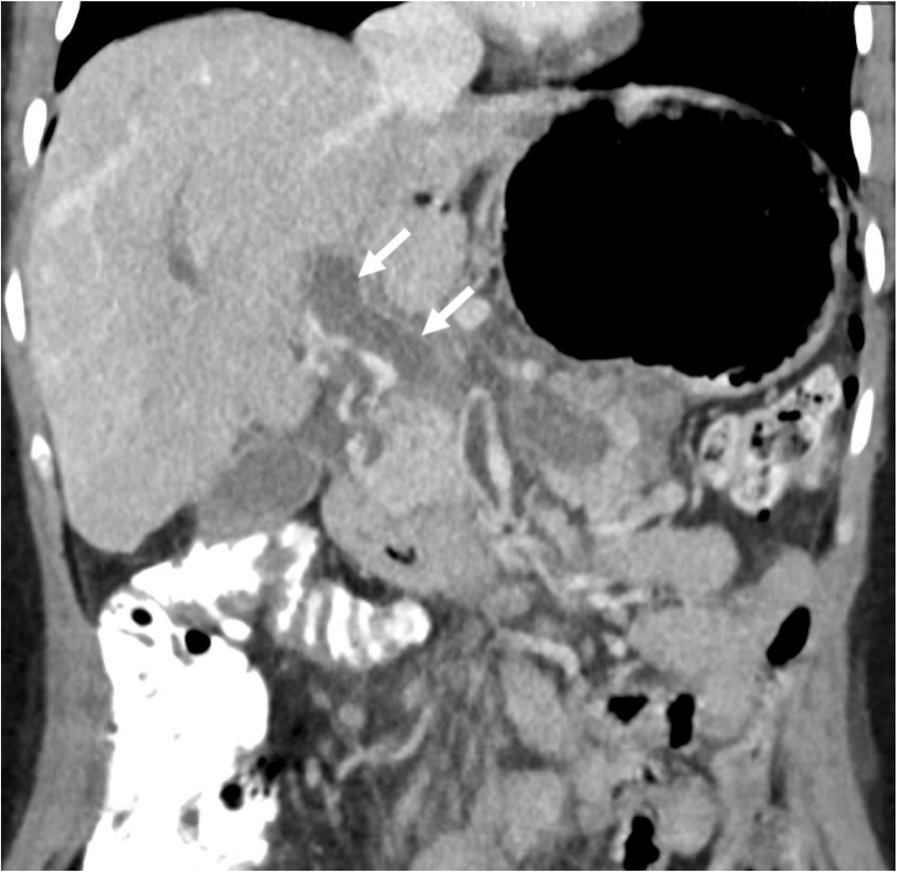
A 34-year-old female with myelofibrosis underwent splenectomy 3 days ago now presenting with right upper quadrant pain and elevated liver enzymes. Coronally reformatted postcontrast CT image demonstrates low attenuating filling defect within the main portal vein (arrows) with no enhancement consistent with bland thrombus
